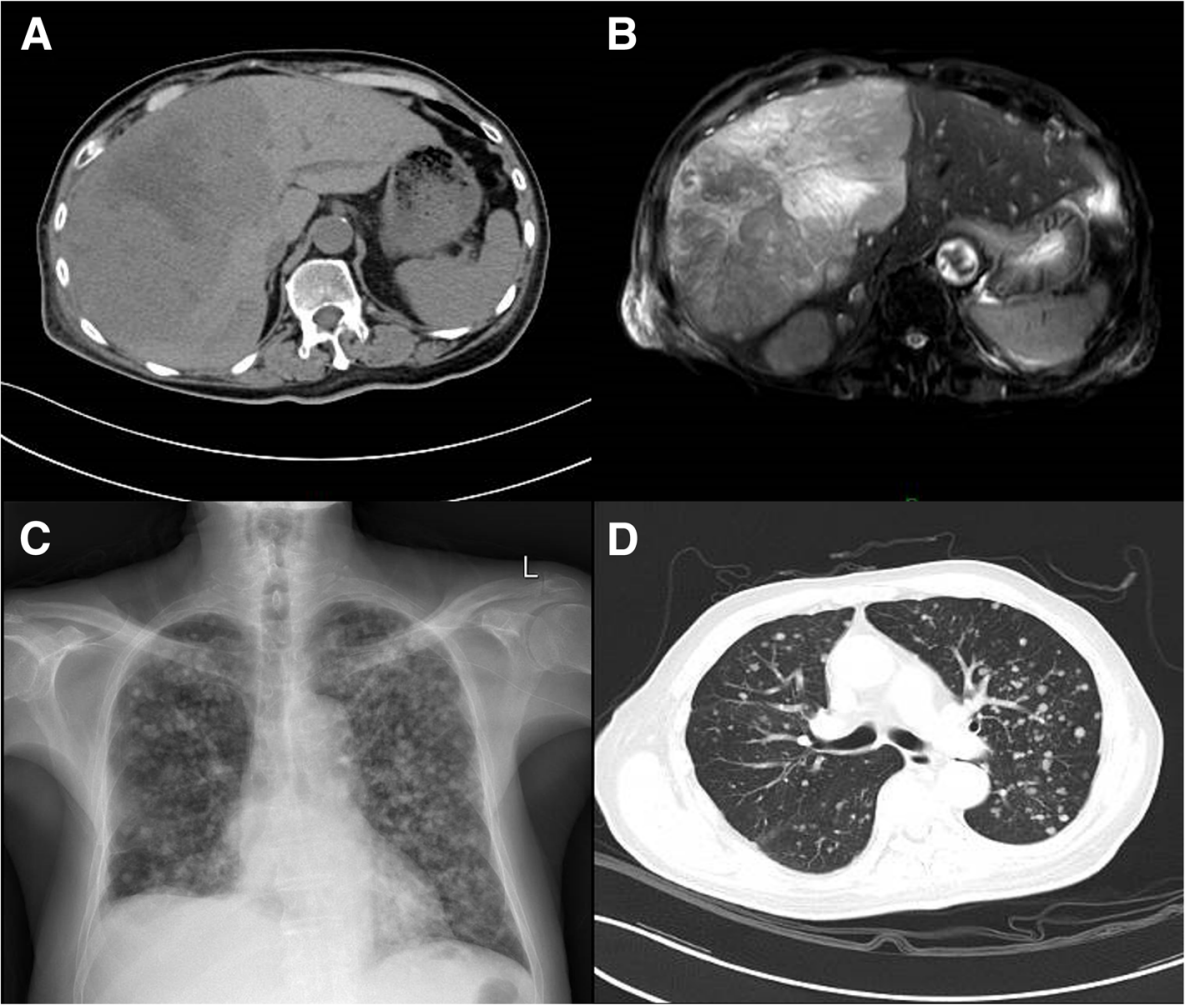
Radiographic images of the involved organs. a CT and b MR image of the primary liver mass. c X-ray and d CT image of multiple metastases to both lungs
